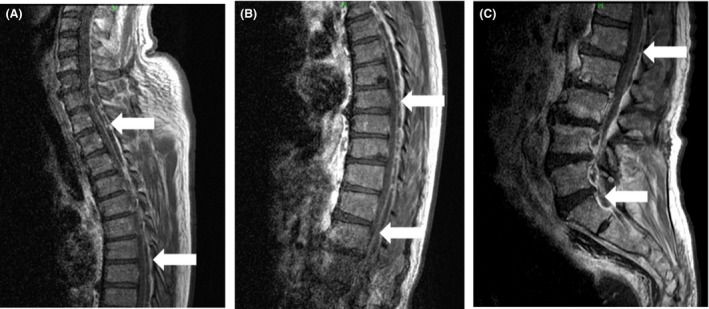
Magnetic resonance imaging with contrast on post‐admission day 4 in a 65‐year‐old male patient admitted with meningeal irritation. A cervical spinal epidural abscess dorsal to the spinal cord extends from C6 (A) (arrows) to the thoracic spine (B) (arrows). The abscess continues to the lumbar spine. Pyogenic spondylitis can be seen from L1 to S1 (C) (arrows).
